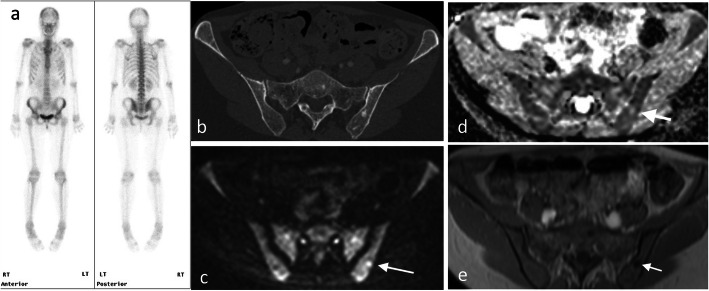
Evaluation of activity of bone disease on bone scan versus WB-MRI with diffusion. Bone scan (a), CT (b) and WBMRI with diffusion (c and d) and T1-W (e) in a 55-year-old woman with breast cancer post-treatment. The bone scan shows no significant abnormality (a), the CT shows foci of bone sclerosis in the pelvis with a visible lesion in the left posterior ilium (b). The lesions demonstrate low signal on the T1-W image (e) and high signal on DWI and intermediate ADC in keeping with disease
